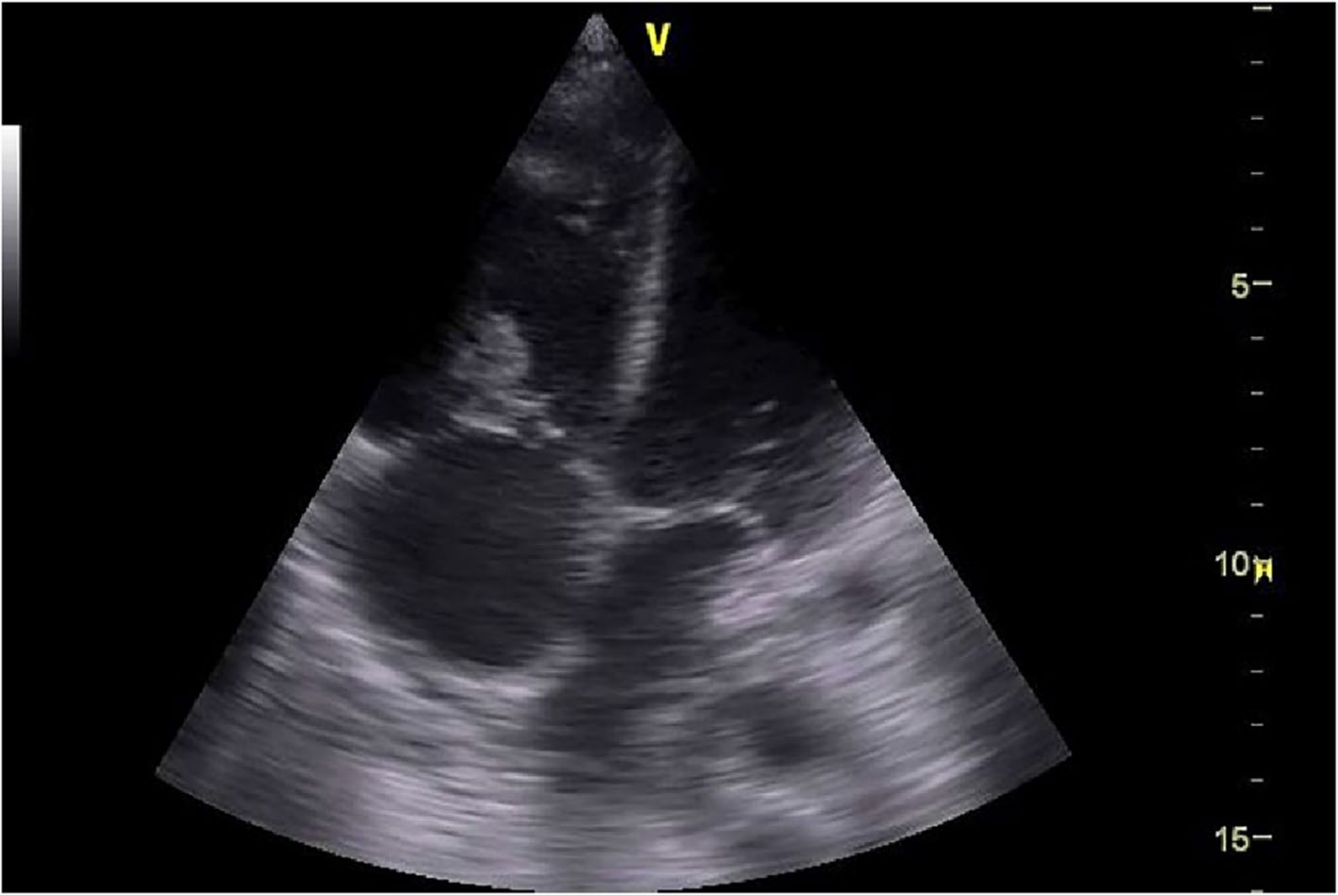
Apical four chamber view showing vegetation on tricuspid valve
